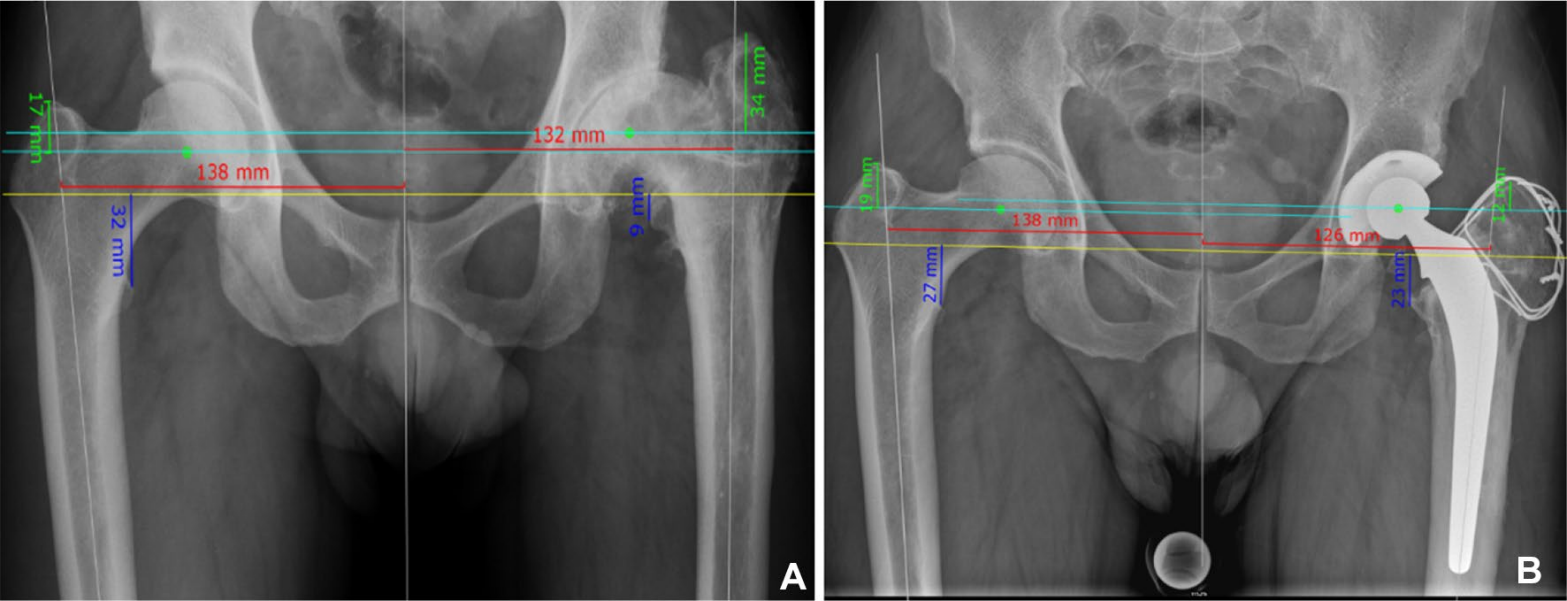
Preoperative (A) and postoperative (B) radiographic assessment of a patient with left-sided sequelae of LCPD. Yellow line: Inter-teardrop line. Red lines: Femoral offset (Sundsvall-method), measured at the height of the most lateral boarder of the greater trochanter as the horizontal distance between the midline of the pelvis and the femoral axis. Green lines: Height of the greater trochanter, measured as the distance between a line through the center of rotation of the hips perpendicular to the inter-teardrop line and the tip of the corresponding greater trochanter. Blue lines: Intraarticular leg length, defined as the perpendicular distance between the inter-teardrop line and the corresponding tip of the lesser trochanter
